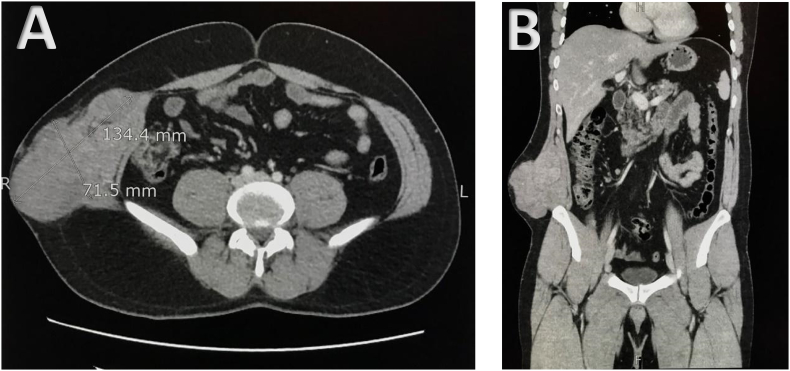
(A-B) Computed Tomography (CT) scan (October 2018) in axial (A) and coronal (B) views showing the primary mass arising from the right lateral abdominal wall.
